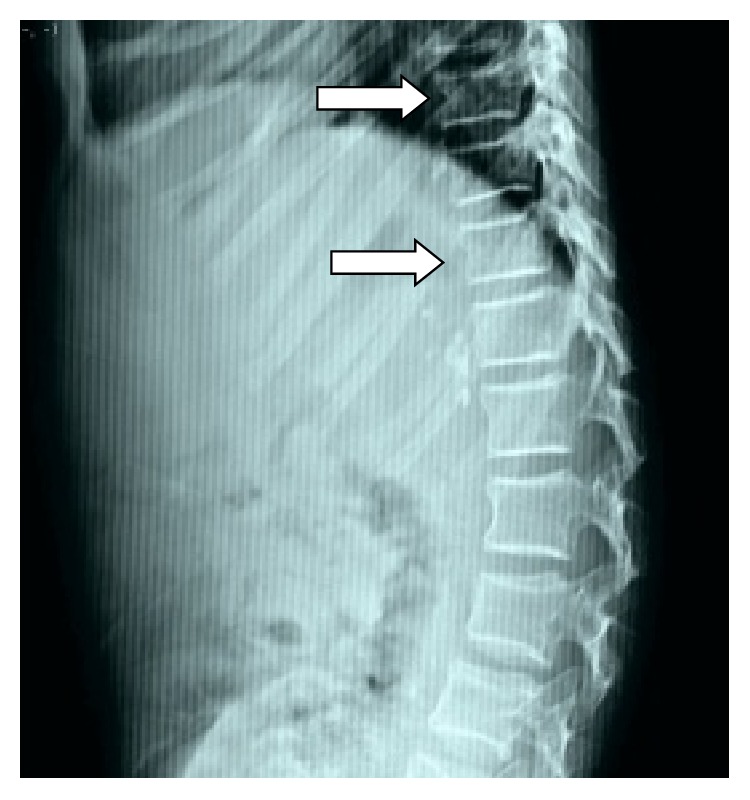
Radiograph of the dorsal spine (lateral projection) showing thoracic kyphosis and mild concavity in the anterior aspect of vertebral bodies (arrows). Prevertebral calcific foci seen adjacent to lower thoracic vertebrae.
